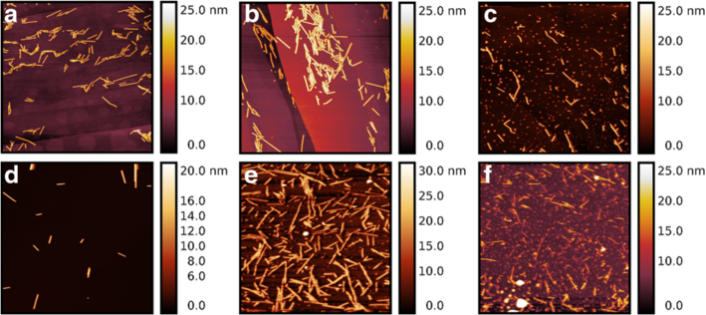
AFM imaging of TMV using tapping mode in liquid environment. *Top row* (a, b, c) is TMV deposited on HOPG whereas *bottom row* (d, e, f) corresponds to TMV deposited on Nickel pre-treated mica. Non-irradiated TMV is shown in (a, d). Increasing exposure to X-ray beam is shown in (b, e) for 10 s exposure, and (c, f) 30 min exposure. The scan size is 5 *μ*m with each line made of 512 pixels. A clear fragmentation of the 300 nm-long TMV can be observed upon increase in exposure time (radiation damage). To the contrary of *β*-Amylase (Fig. 2), even at standard exposure time, a beginning of fragmentation is observed for TMV. A total of 14 AFM images has been used for statistical analysis representing 6539 particles on HOPG at 10 *μ*m scan size, 2007 particles on HOPG at 5 *μ*m scan size, and 808 particles on nickel pre-treated mica
